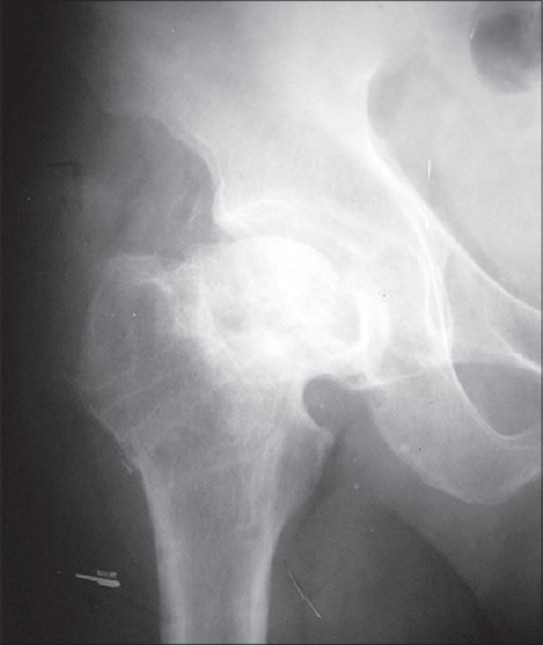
X-ray antero posterior view showing avascular necrosis and collapse of femoral head following primary valgus osteotomy and osteosynthesis, at two years follow-up
